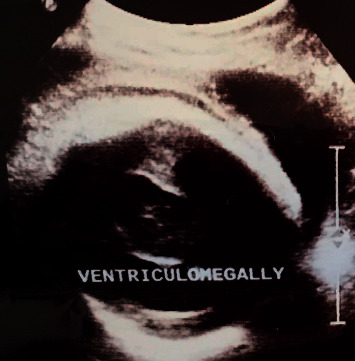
Antenatal ultrasound scan of the fetal head showing a single dilated ventricle with a considerable cortical mantle and a fused thalamus with completely fused hemispheres but without septum pellucidum.
